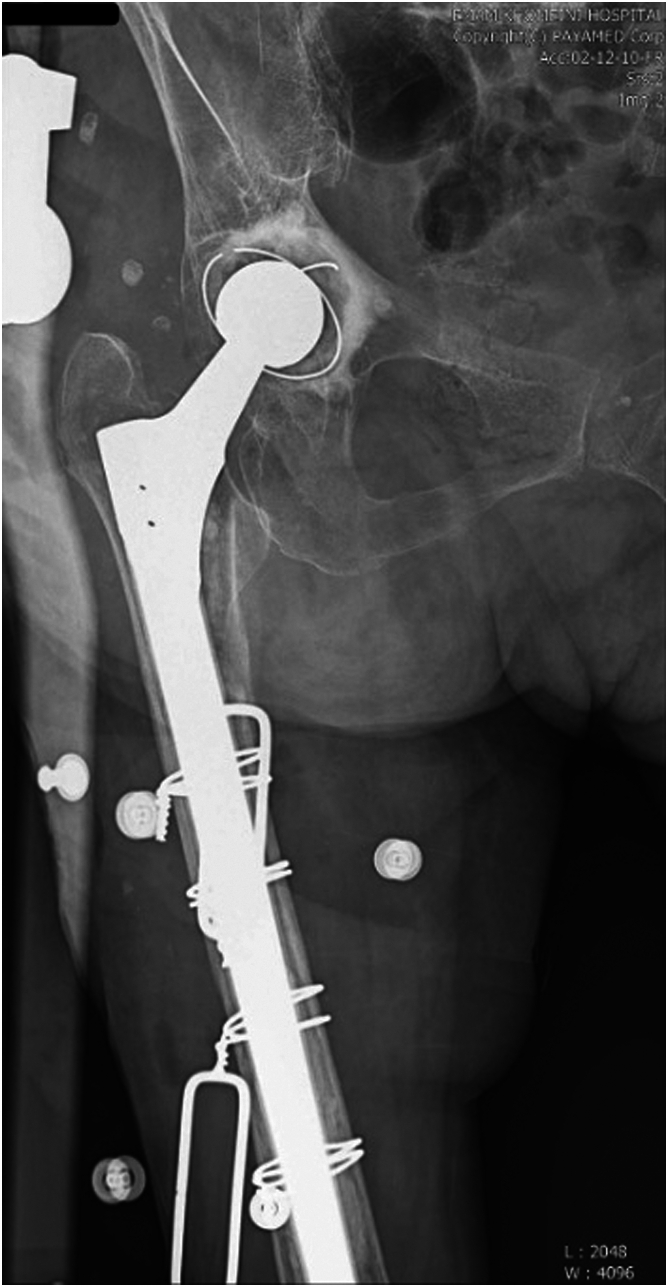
Postoperative radiograph of case 1.
